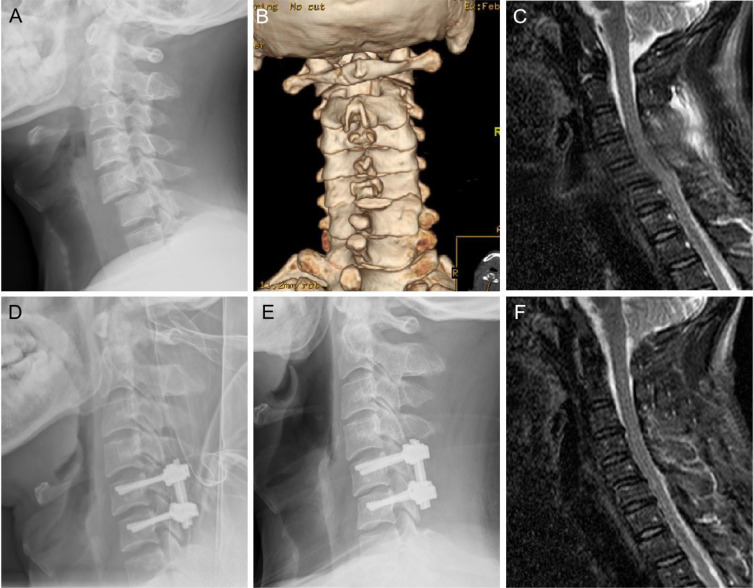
Imaging of posterior surgery. A 41-year-old man with C5/6 dislocation (A). 3D-CT showed the right facet was interlocked (B). X-Ray showed satisfactory reduction (D). Narrowing of intervertebral space and loss of the lordosis angle in C5/6 level at 14 years after surgery (E). MRI showed no spinal stenosis in C5/6 level, and the spinal cord signal was no abnormal (F).
